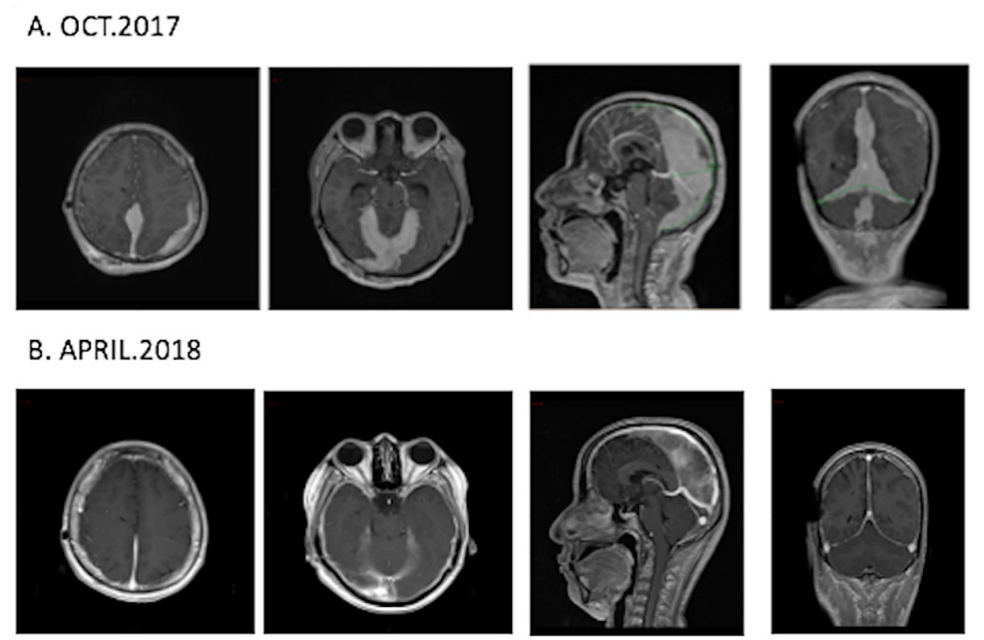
Comparative brain MRI (A) Brain MRI (October 2017): extensive infiltrative involvement of multiple portions of the dura mater, particularly in supra and infratentorial interhemispheric regions and in the tentorium. Additional lesions at the diploe level are present, highly suggestive of a hematogenous neoplasm with extranodal involvement. (B) Brain MRI (April 2018): radiological evolution of primary CNS lymphoma following external radiotherapy from December 11, 2017, to January 03, 2018: WBRT 36 Gy/2Gy with evidence of dura mater infiltration compatible with partial response, with signs of neoplastic infiltration persistence in the tentorium and in the posterior region of the brain sickle.
